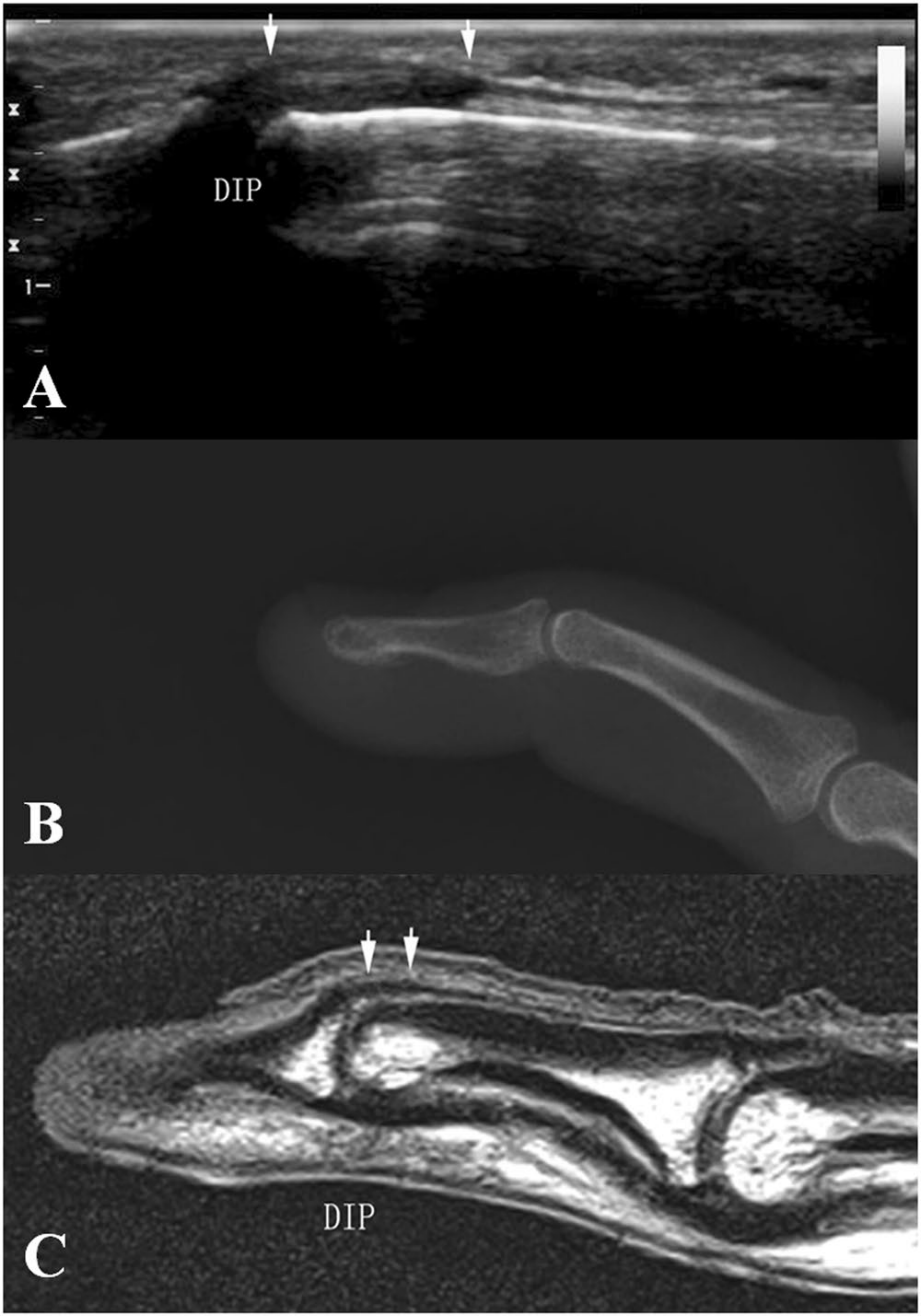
Type C mallet finger. High frequency ultrasonography (Fig. 4A), X-ray (Fig. 4B) and MRI (Fig. 4C) images of type C mallet finger injury. The injured extensor tendon (arrow).
